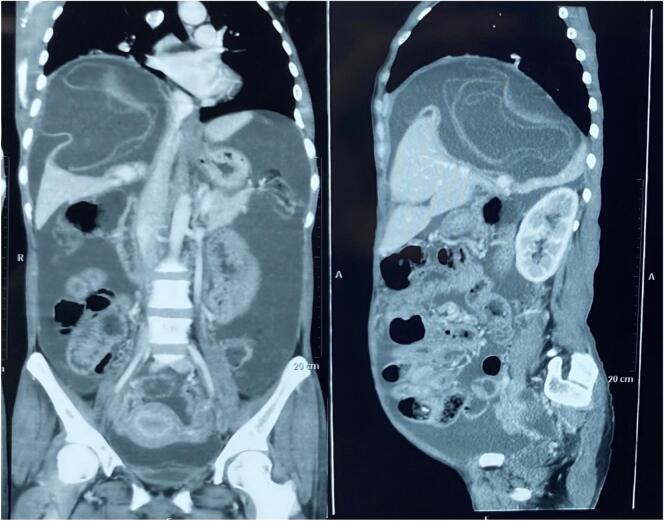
CECT Abdomen: Coronal (A) and Sagittal (B) section showing ruptured hydatid cyst of the liver with collection in the peritoneal cavity. CECT: Contrast Enhanced Computed Tomography.
